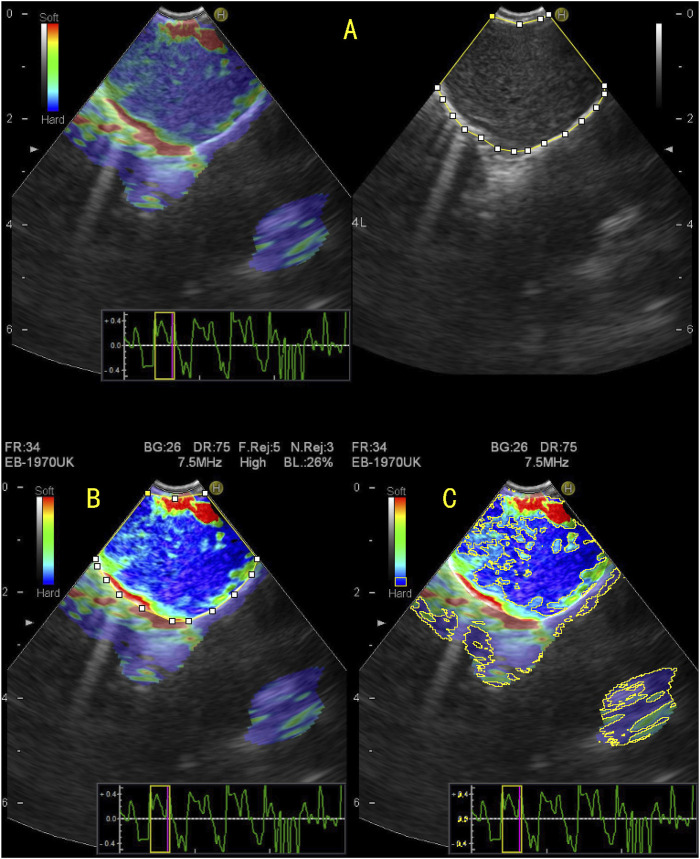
Quantitative evaluation of endobronchial ultrasound elastography using ImageJ. (A) The elastography image on the right and the B-mode ultrasound image on the left, on which the entire area of the target lymph node was manually enclosed; (B) To enhance the color contrast between the target lymph node and surrounding tissue; (C) By adjusting the blue value, brightness, and saturation, the blue region in the target lymph node is displayed and the area of the blue region in the target lymph node is measured. The stiffness area ratio (SAR) was calculated by dividing the blue area (indicating stiffness) by the entire area of the lymph node. SAR = 0.624, pathological diagnosis was malignant.
